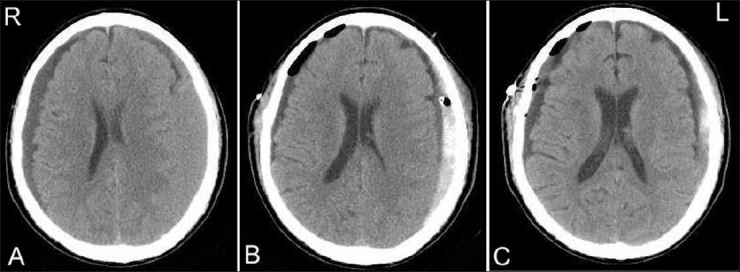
CT images of case 4. (A) CT scan on admission, showing bilateral CSH. (B) CT scan 1 day after the surgery, showing a new hemorrhage in the left side of hematoma cavity. (C) CT scan 2 weeks after the surgery, showing an increase in left subdural hematoma
